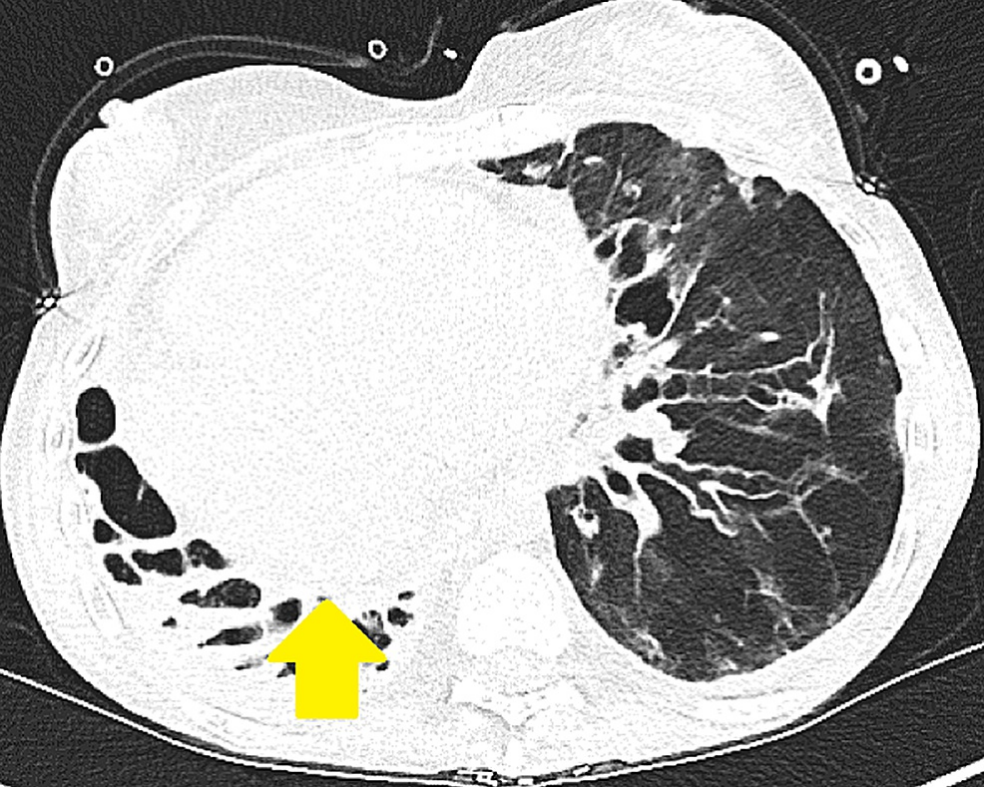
CT chest axial view shows bronchiectasis with extreme right-sided deviated mediastinal structures (yellow arrow)
